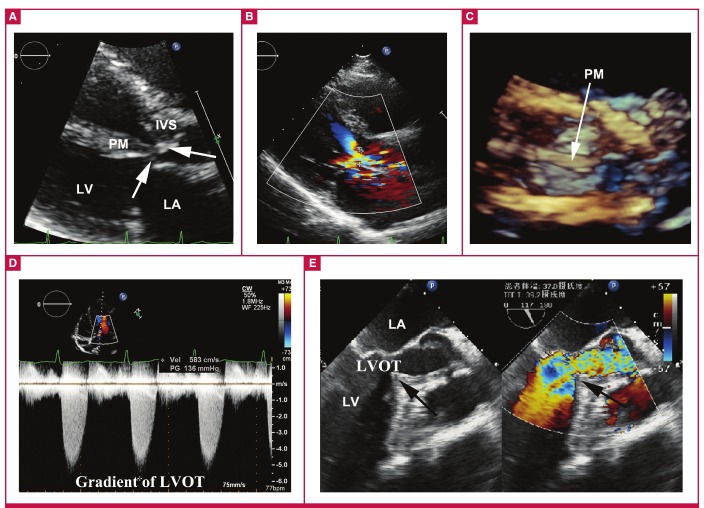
LVOT obstruction due to basal IVS hypertrophy (A) and PM malposition (A, C). B shows the narrowed blood flow of the LVOT. Pulsed Doppler demonstrated a gradient of 136 mmHg (D). Intra-operative transoesophageal echocardiography (E) showing the sharp angle of the IVS (black arrow).
